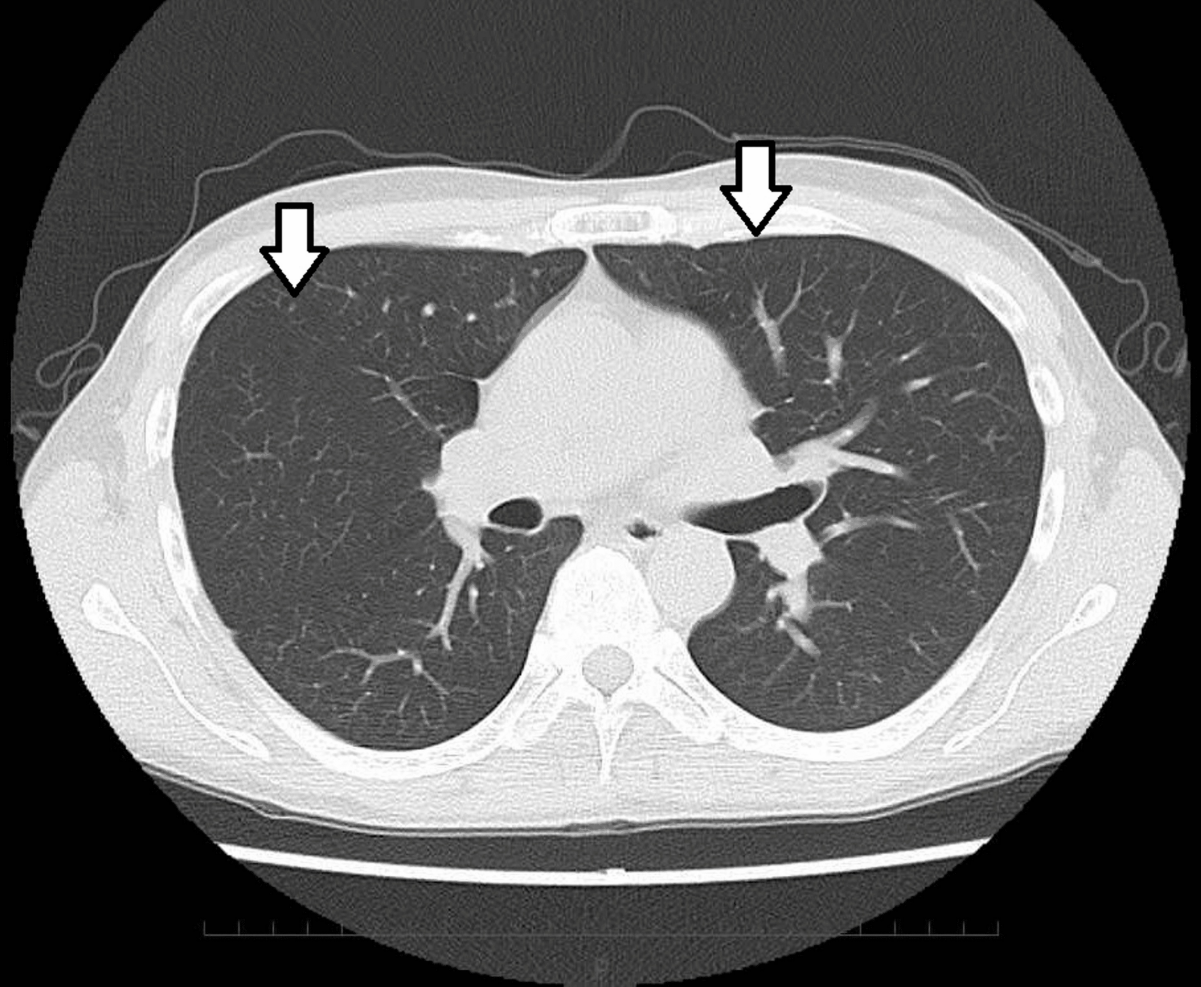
CT scan at first follow-up after two cycles of EV showing complete response with resolution of all lesions (white arrows). EV: enfortumab vedotin
